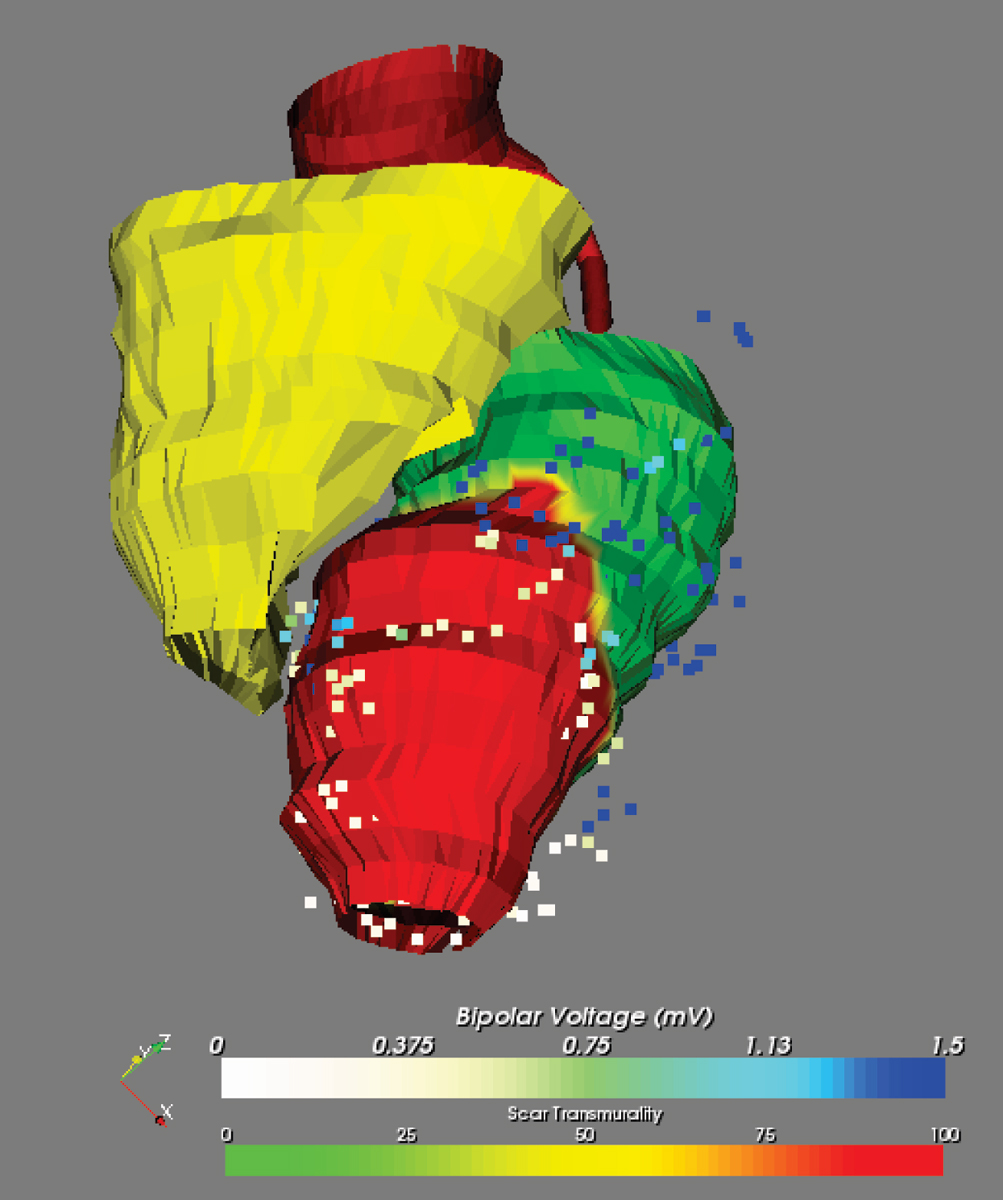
MRI derived surface mesh depicting the LV endocardial (color coded), RV endocardial (in yellow), aorta (in red) and RCA (in red) anatomy. Coloring of the LV endocardial surface is used to indicate the transmural extent of scar in the endocardial layer of the myocardium (green = 0%, red = 100%). The square dots indicate the mapping locations obtained during the VT ablation procedure. Color coding is used to indicate the bipolar electrogram amplitude (white ≤ 0 mV, dark blue ≥ 1.5 mV).
